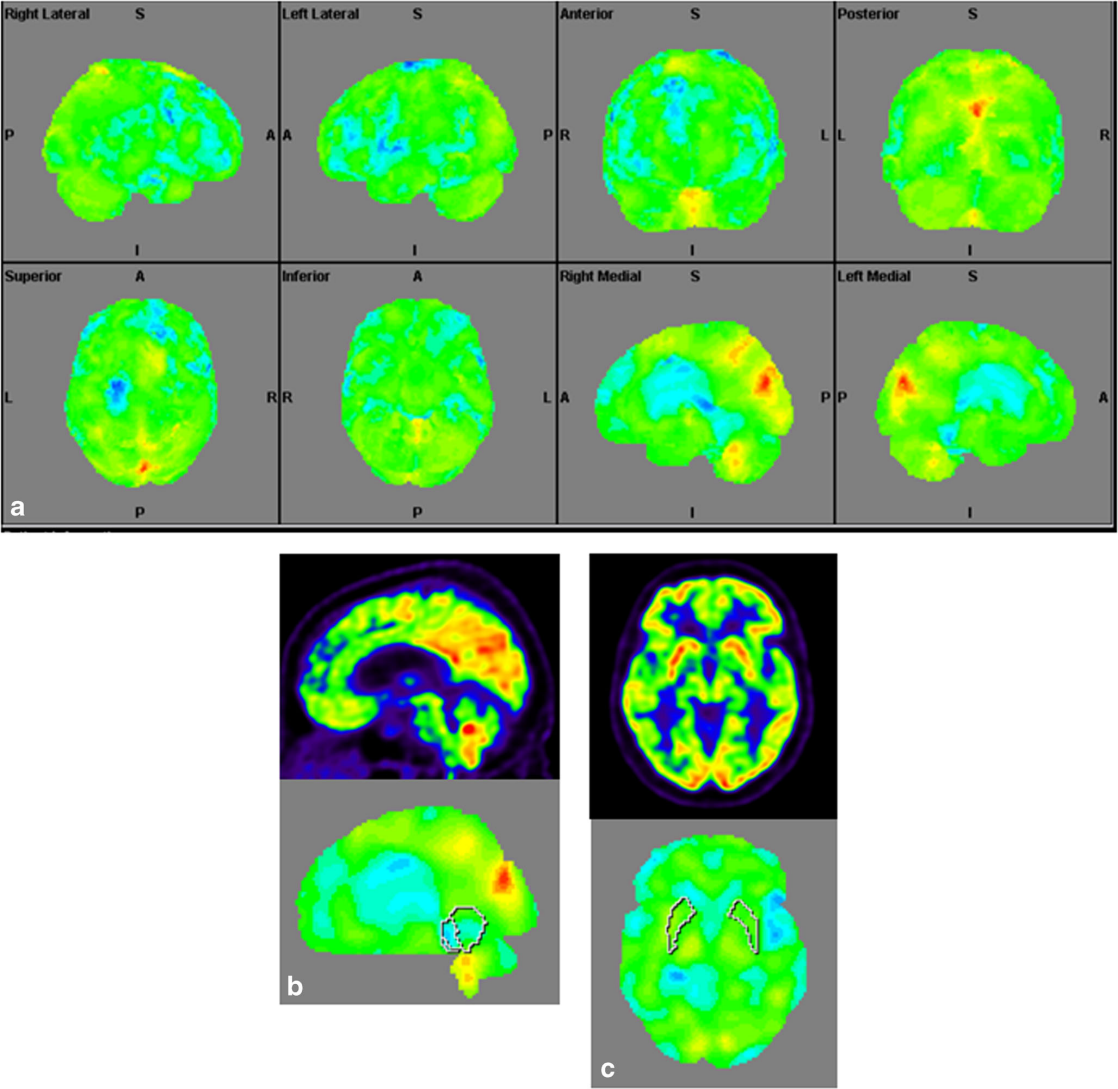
Surface projections (3DSSP) of brain [^18^F] FDG PET findings for patient III:2. a Widespread hypometabolism in the prefrontal cortex and some areas of the motor and temporal cortices is evident. Metabolism is also reduced in the vermis but normal in the cerebellar hemispheres. b Midsagittal image shows reduced FDG uptake in the vermis (upper case) as compared to the reference values in VOI templates (lower case). c Coronal image shows normal FDG uptake in the putamen (upper case) as compared to the reference values (lower case)
